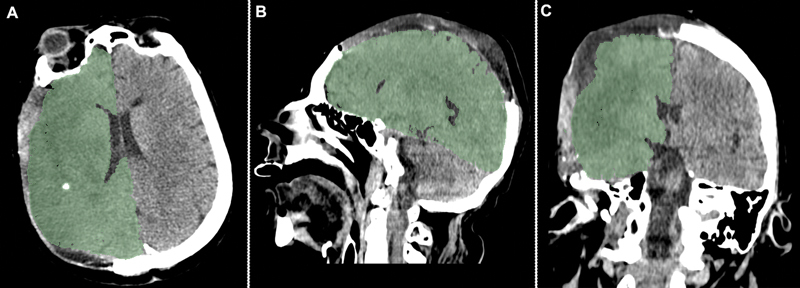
The light green area represents the segmented right hemisphere after decompressive craniectomy, in a postoperative computed tomography exam.
